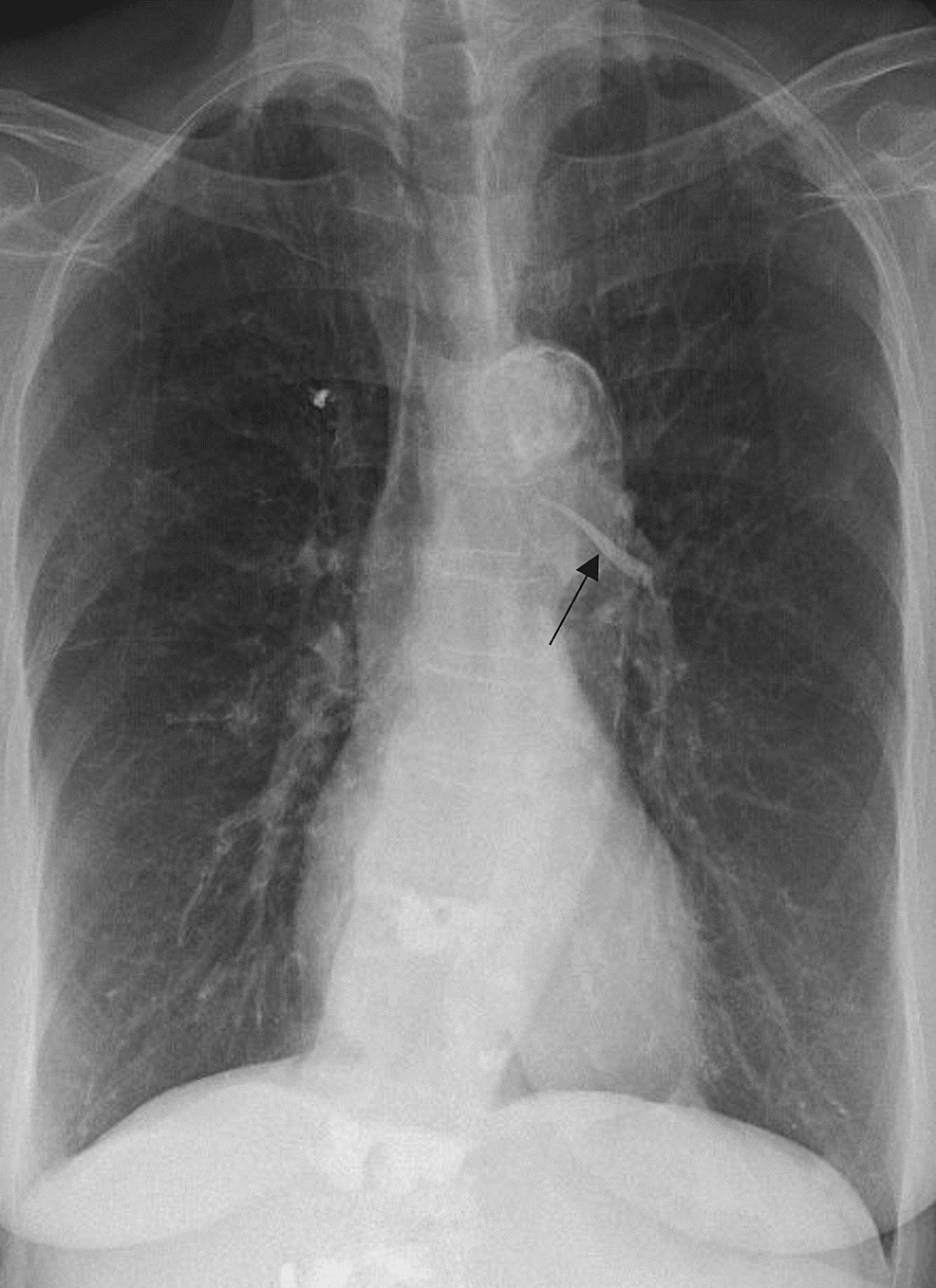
Admission chest x-ray Chest x-ray demonstrating high-density material in the pulmonary vasculature likely reflecting embolized cement is demonstrated by the black arrow.
